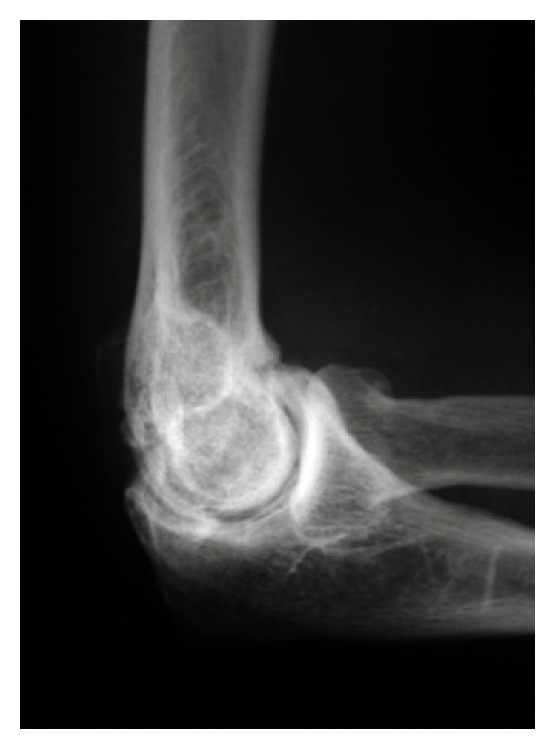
Lateral radiograph demonstrates idiopathic arthritis with osteophytes on the radial head as well as anterior and posterior ulnohumeral joints.
